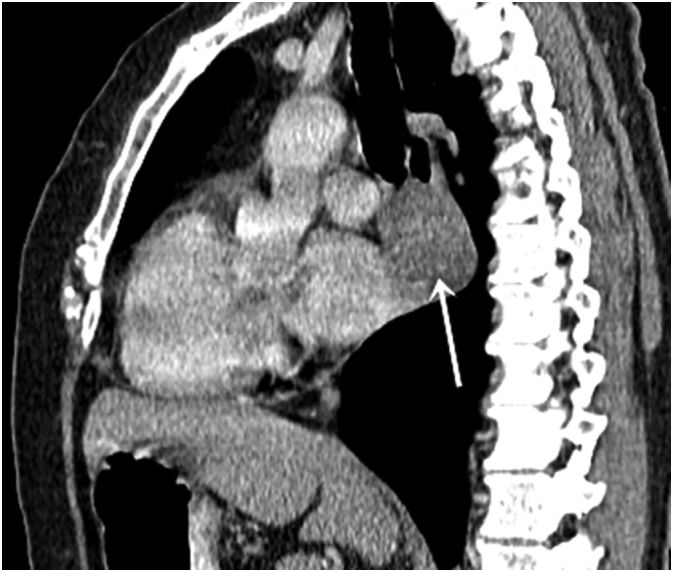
CT scan in the sagittal plane showing a moderately enhancing mediastinal mass displacing the esophagus in its middle third (white arrow).
